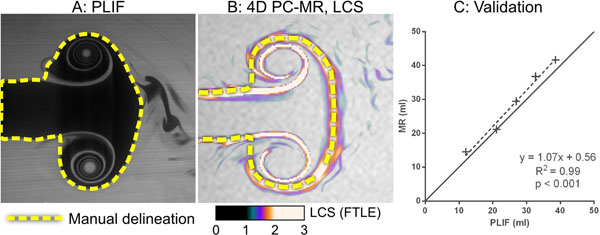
Data analysis in PLIF and 4D PC-MR images and quantitative results for vortex ring volume (VV). Panel A: Analysis of PLIF images. Panel B: Analysis of 4D PC-MR data using Lagrangian Coherent Structures (LCS). Panel C: Comparison of vortex ring volume (VV) at the five pump settings.
